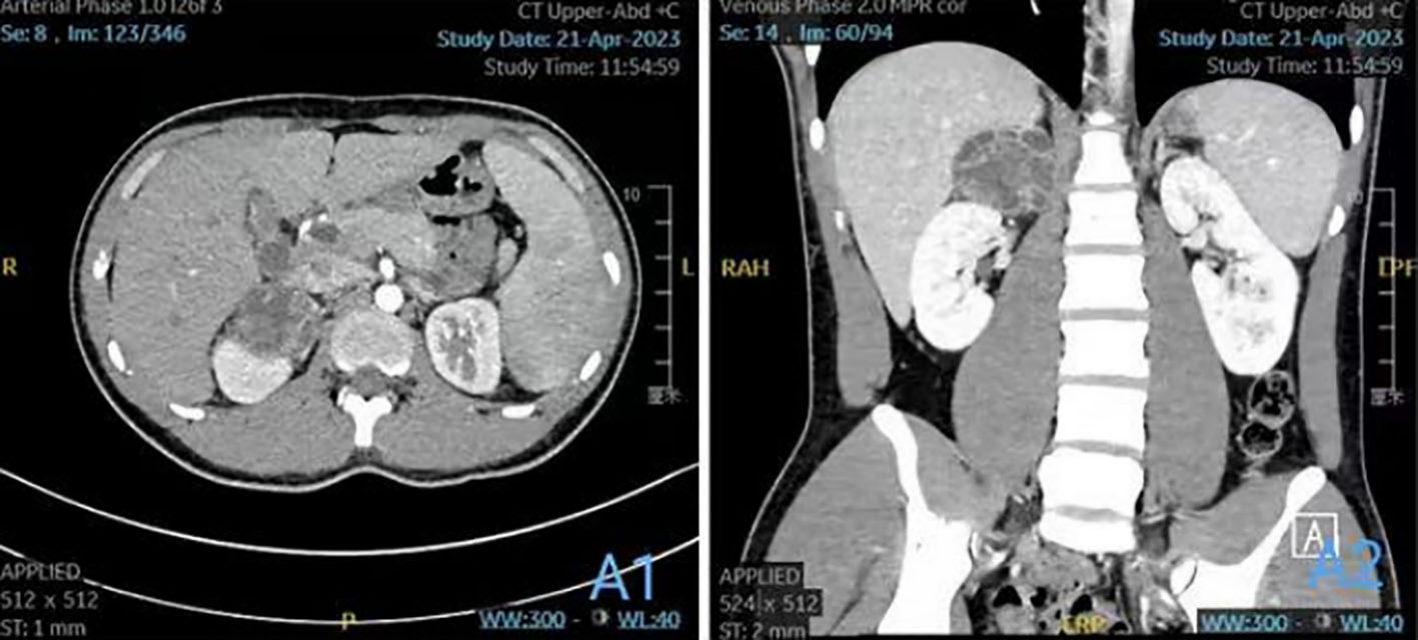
(A1) Plain and enhanced CT scan of the upper abdomen; (A2) coronal image.
